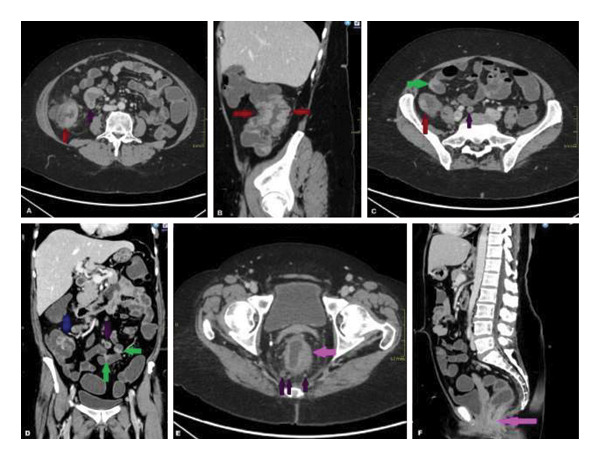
(A) Axial, post contrast CT showing eccentric noncircumferential enhancing mural thickening of the ascending colon (red arrow) along the mesenteric border with surrounding mesenteric fat stranding. Also shown is a conglomerate necrotic nodal mass (purple arrow) in the right iliac fossa is an ileocolic node; (B) sagittal contrast‐enhanced CT, again shown is the mural thickening and enhancement of the ascending colon (red arrows); (C) thickening of the ascending colon again shown (red arrow). Noncircumferential enhancing mucosal lesions of the distal ileum (green arrow) depicted in the right iliac fossa. Also shown is a necrotic right common iliac node (purple arrow); (D) coronal CT images showing mural thickening at the ileocecal valve (blue arrow). Multiple enhancing mucosal lesions in the mid ileum (green arrows) and nodes (purple arrow) are shown; (E, F) left eccentric hemi‐circumferential mural thickening of the low rectum (pink arrow) most prominent at 1 to 6 o’clock with mesorectal fat stranding and prominent mesorectal nodes.
